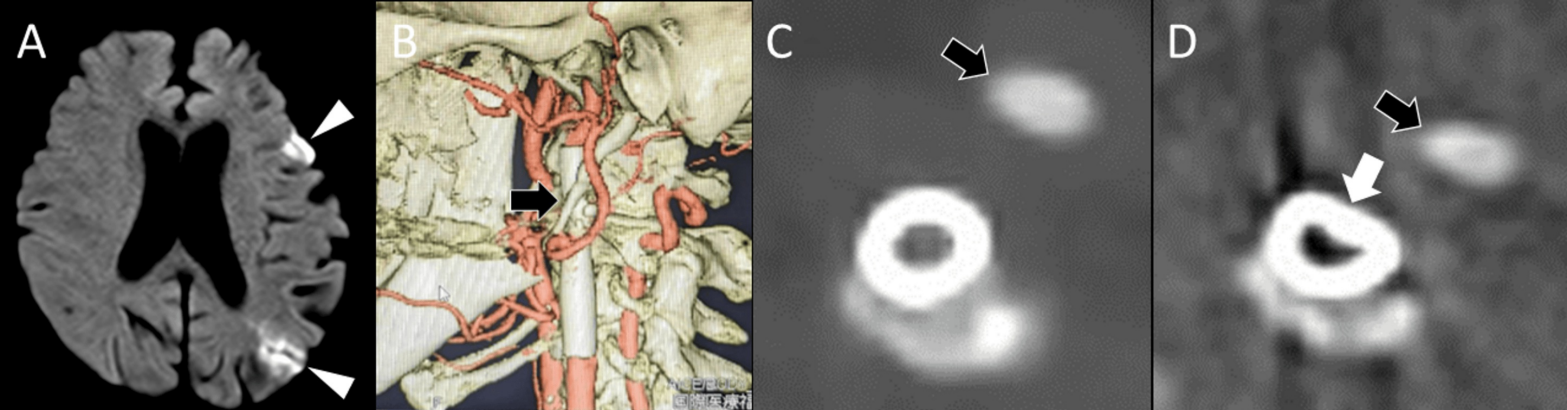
Imaging at the time of stroke recurrence. (A) Diffusion-weighted MRI showing recurrent cerebral infarction in the left watershed area (arrowheads). (B) Three-dimensional CT angiography (3D-CTA) revealing a close proximity between the carotid stent and the elongated styloid process (black arrow). (C) Post-carotid artery stenting CT showing no stent deformation immediately after the procedure. (D) At the time of stroke recurrence, the stent adjacent to the styloid process (black arrow) was deformed (white arrow).
